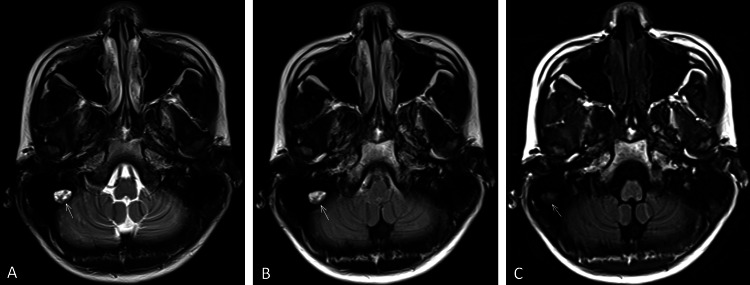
Characterization of the artifact in Case 1 (A) Axial T2 imaging and (B) axial T2FLAIR demonstrate an oval-shaped, well-circumscribed hyperintense artifact (arrows) in the right mastoid region. (C) On Axial T1 imaging, the artifact appears isointense is positioned slightly more lateral compared to its localization on T2 and T2FLAIR sequences (arrow). FLAIR: Fluid-attenuated inversion recovery.
